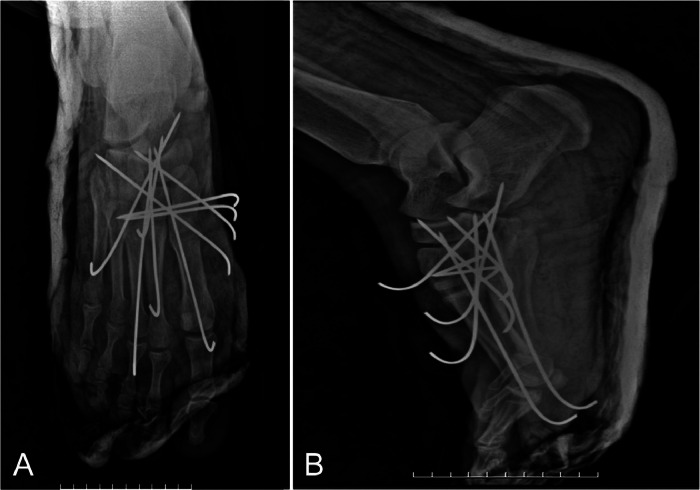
Plain radiographs of the right foot taken 1 day after surgery. (A) Anteroposterior (AP) view, (B) Lateral view. K-wire fixation and closed reduction were performed, and the medial cuneiform and metatarsals are in normal alignment.
